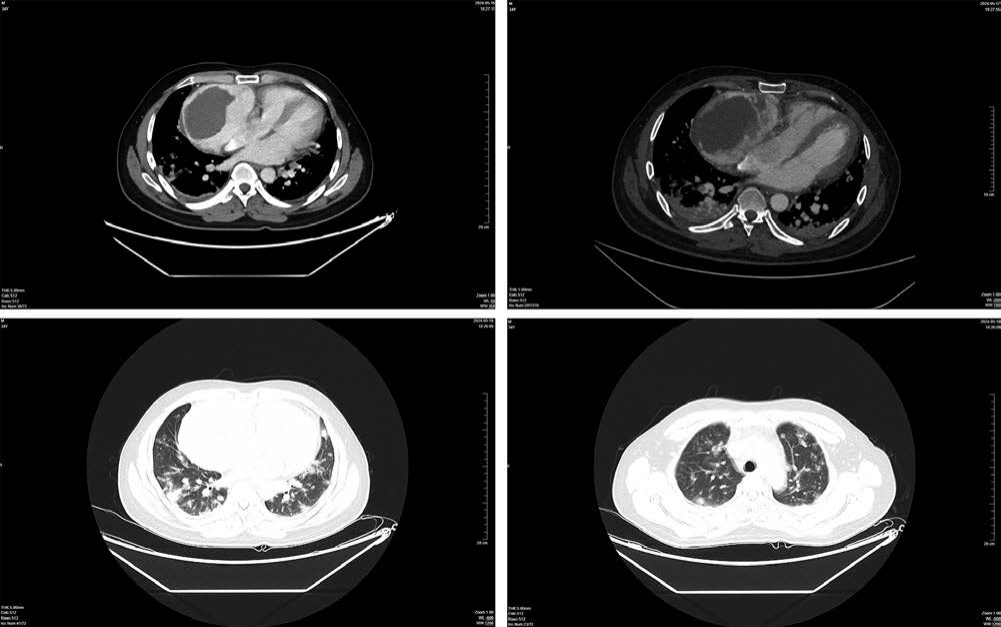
Chest computed tomography (CT) scan.
